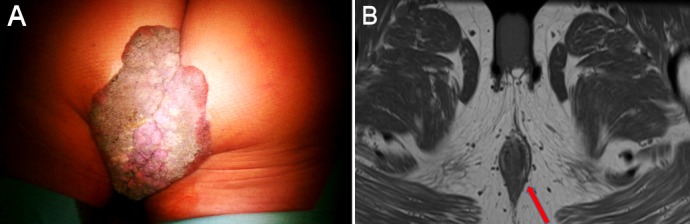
A) Preoperative aspect of the lesion (16 x 12 cm). B) Preoperative MRI: Suspect infiltration.
